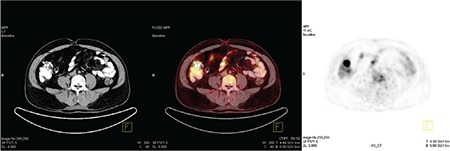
Markedly increased ^18^F-FDG uptake is seen in soft tissue lesions of the colonic hepatic flexure, which was the largest lesion in this patient.
